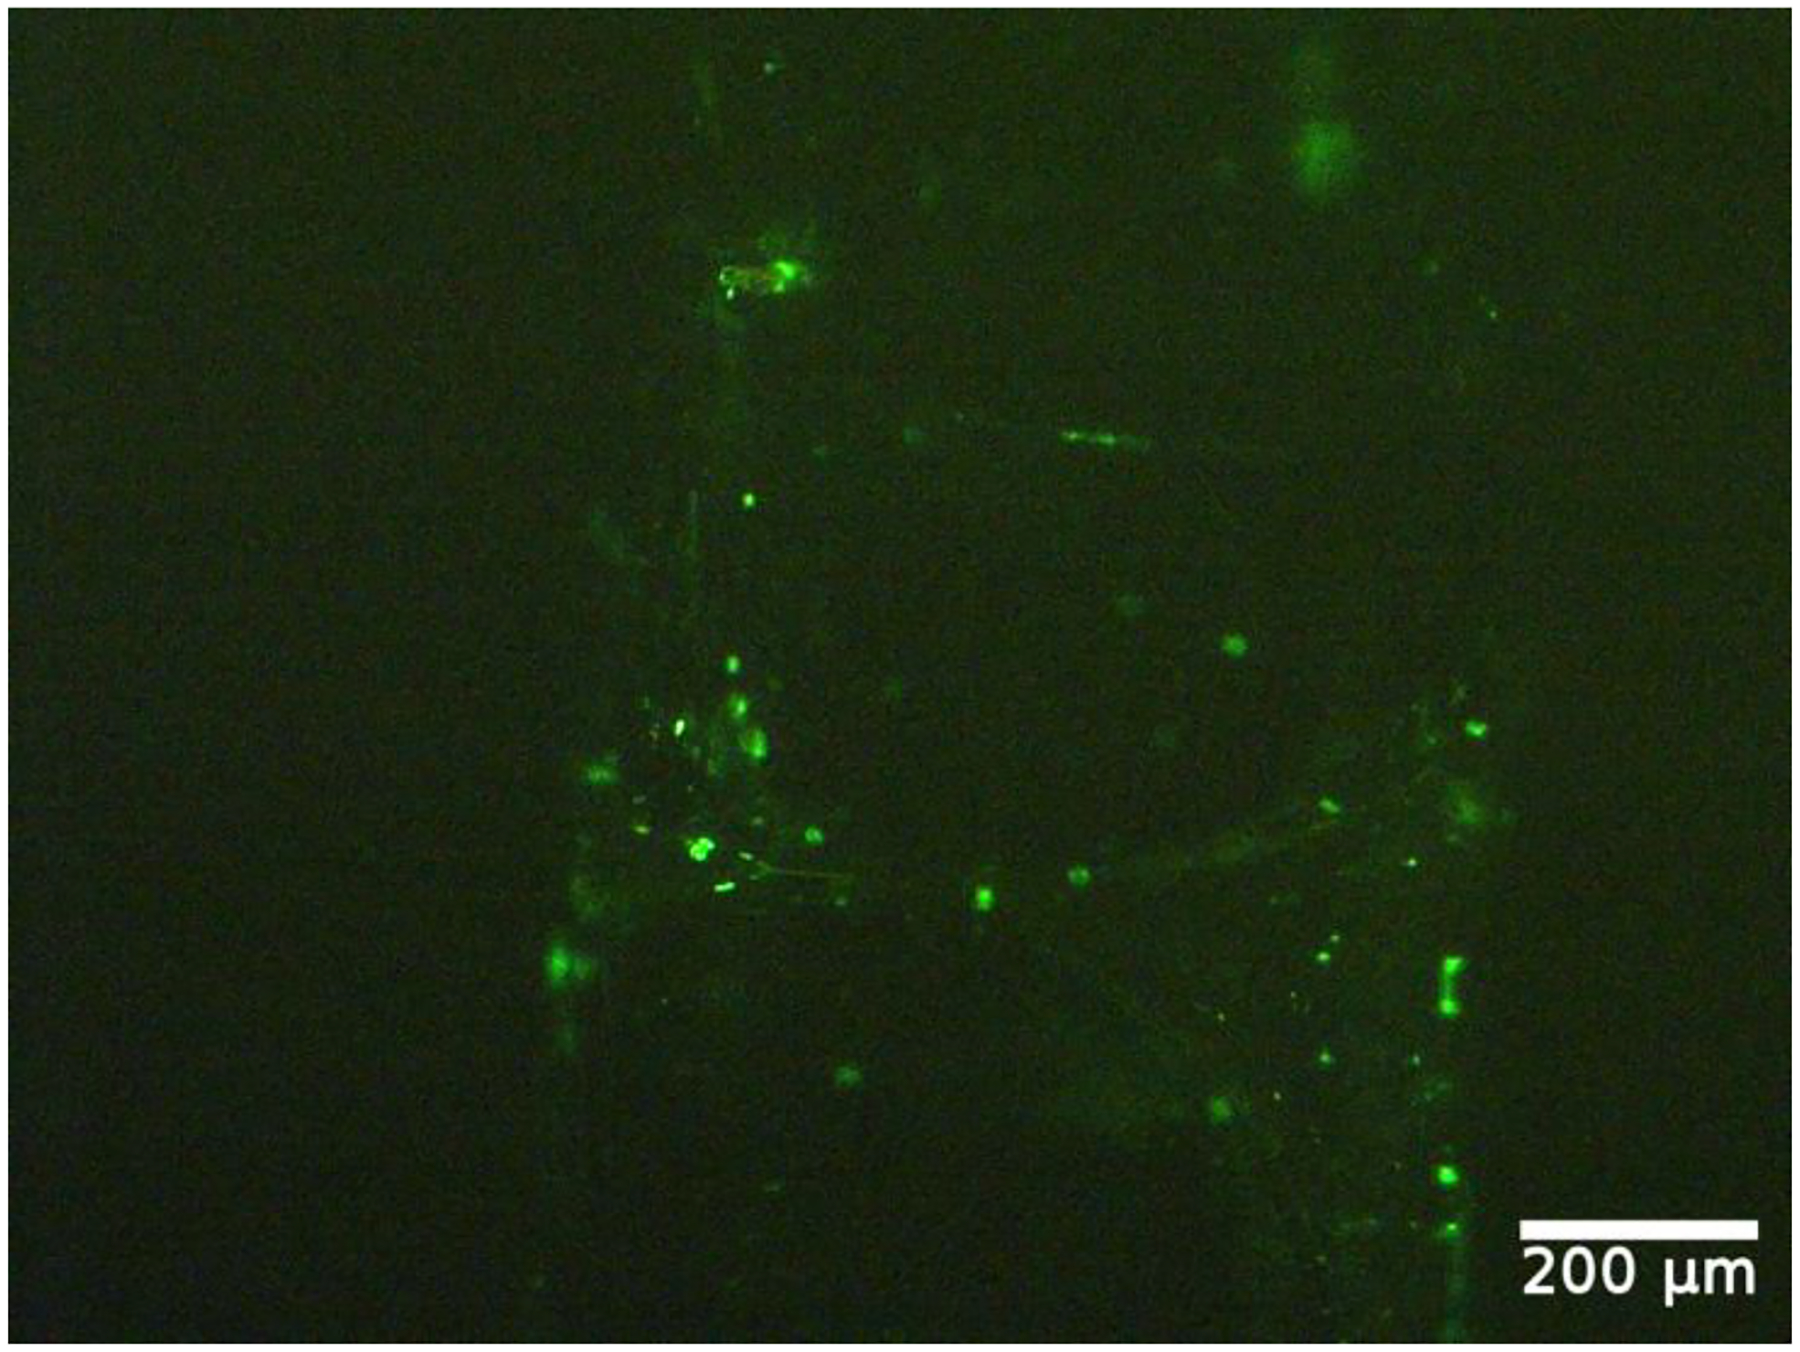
Apoptosis of endothelial cells after being treated with 0.5 MPa ultrasound peak negative pressure and 15 mJ/cm^2^ laser fluence. Apoptotic cells show green fluorescence, and live cells show little or no fluorescence.
